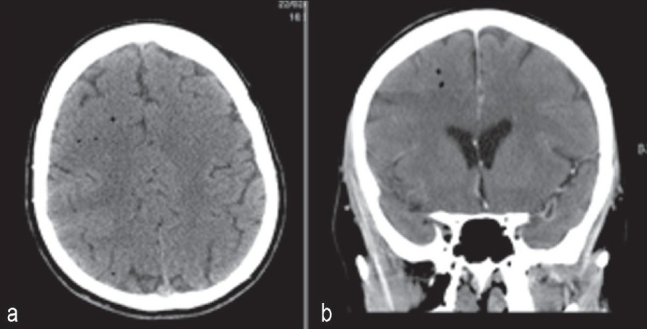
(a) CT head showing air bubbles predominantly in the right cerebral hemisphere (b) CT head showing air bubbles predominantly in the right cerebral hemisphere
